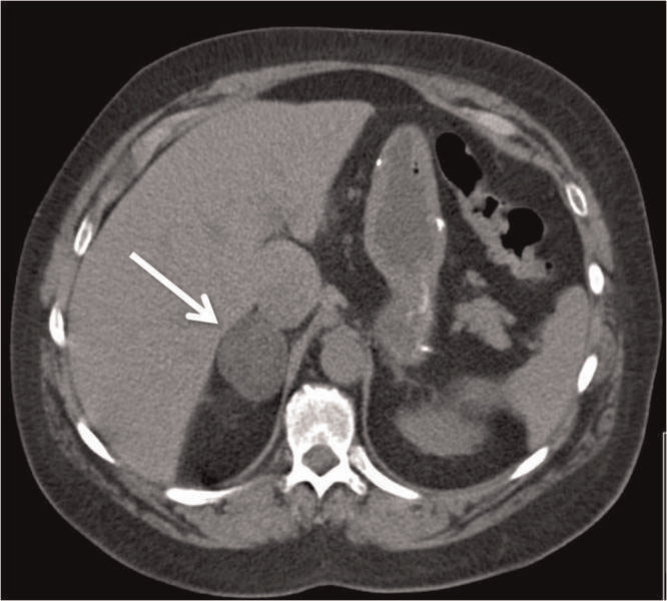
Abdominal CT scan, arrow depicts right adrenal mass.
